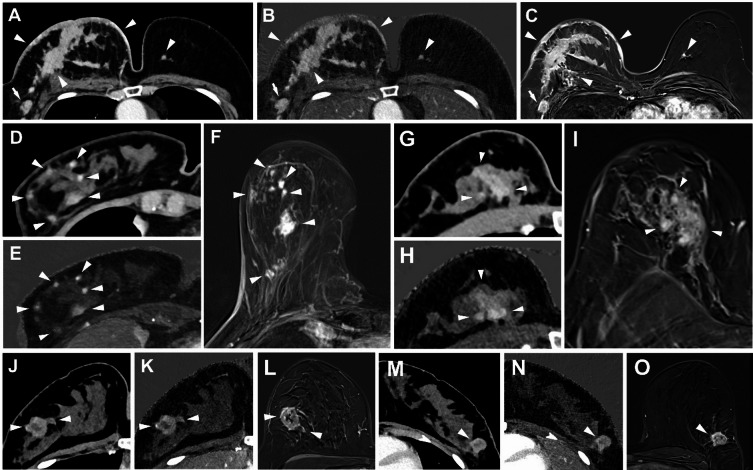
61-year-old participant with bilateral no special type right-sided Luminal B HER2 + G2 and left-sided Luminal B HER2- G1 breast cancer (arrowhead, A-C) and right-sided axillary lymph node metastasis (arrow) (A-C); 63-year-old participant with right-sided multifocal HER2 type G2 cancer (arrowheads, D-F), and 52-year-old participant with right-sided bifocal Luminal B HER2- G2 breast cancer (arrowheads, G-I), both of no special type associated with DCIS, and 32-year-old participant with bilateral unifocal triple negative G3 cancer with central necrosis (J-O). Shown are monoenergetic 65 keV reconstructions (A, D, G, J, M) and iodine maps (B, E, H, K, N) of contrast-enhanced thoracic photon-counting detector computed tomography, and subtraction images of contrast-enhanced MRI (C, F, I, L, O). Whilst the cancer of the first participant on the right side is clearly detectable but difficult to be measure accurately in all modalities due to diffuse expansion with dermal and pectoral muscle invasion (arrowheads), the left-sided cancer is clearly visible and exactly measurable despite its small size (arrowhead). In the second and third cases multifocality can clearly be seen but again cancer size is difficult to be accurately measured due to accompanying DCIS. In the last case (J-O), triple negative cancer is clearly visible and measurable in both breasts.
